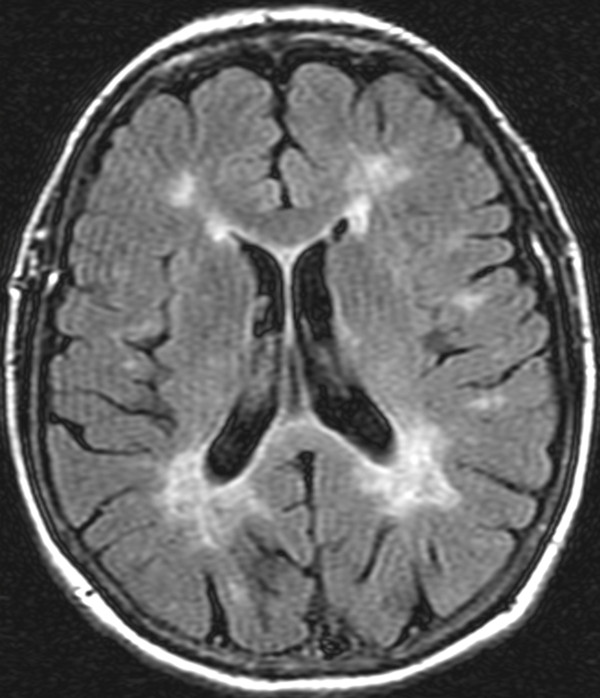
Magnetic resonance imaging scans: fluid attenuated inversion recovery (FLAIR) sequence, transverse plane. Shown are demyelinating areas involving the periventricular areas of the lateral ventricles, more prominent in the posterior. A similar demyelination process is present in the white matter of the brain sulci (medullary white matter).
